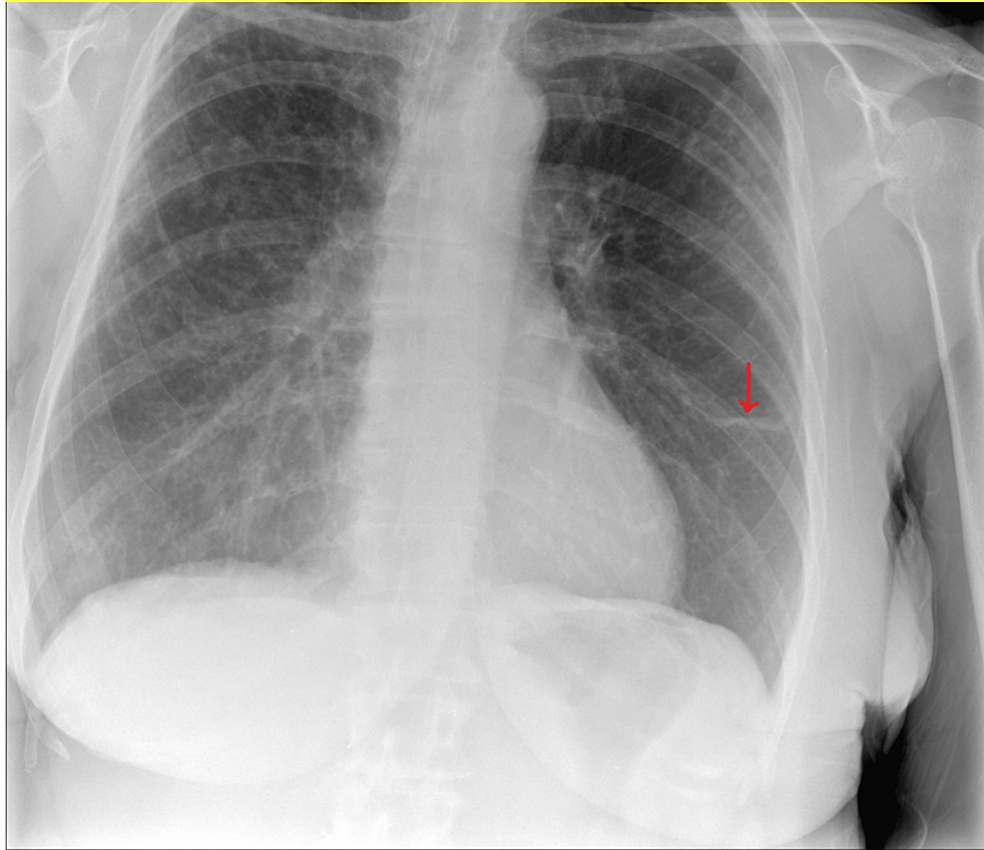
Chest X-ray showing bilateral abnormal shadowing Minimal abnormal shadowing can be seen in the right mid- and upper zones and the left mid-zone. The red arrow points to a small area of atelectasis in the left lower zone.
